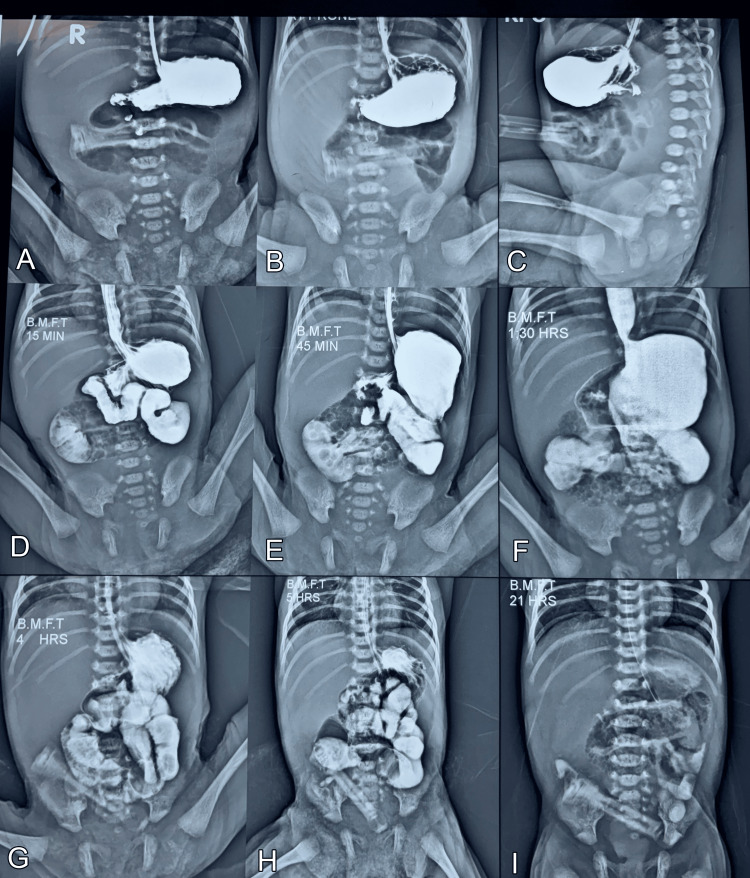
Barium meal follow-through contrast study. This series of radiographic images shows the transit of barium contrast medium through the gastrointestinal tract over a 21-hour period. Supine (A,B) and lateral (C) baseline views prior to the follow-through, showing contrast in the esophagus and stomach. (D) At 15 minutes, contrast has entered the small bowel loops. (E) By 45 minutes, there is progressive filling of the distended proximal small bowel. (F) At 1 hour and 30 minutes, large, dilated small bowel loops in the upper abdomen are filled with contrast. (G) By four hours, there is persistent, severe dilation of the small-bowel loops in the mid-abdomen. (H) At five hours, significant contrast stasis remains in the extensively dilated small bowel. (I) At 21 hours, a small amount of residual contrast is seen in the colon, but marked small bowel dilation is still present, indicative of a severe, chronic-type obstruction
